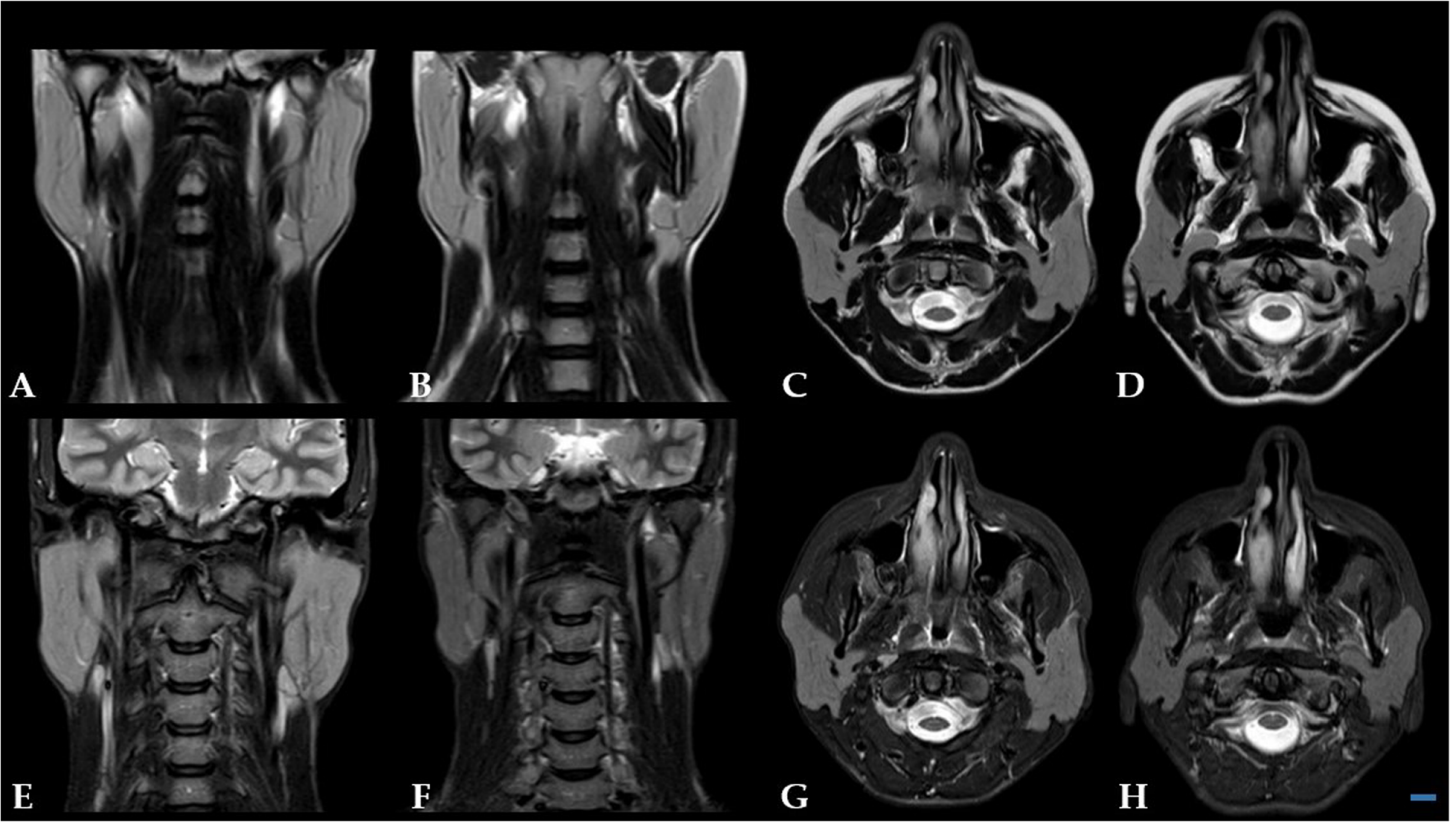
MRI assessment shows reduction of the parotid gland volume bilaterally. Coronal T2w at T0 (a) and T1 (b); axial T2w at T0 (c) and T1 (d); coronal STIR at T0 (e) and T1 (f); axial STIR at T0 (g) and T1 (H). BAR: 1 cm
